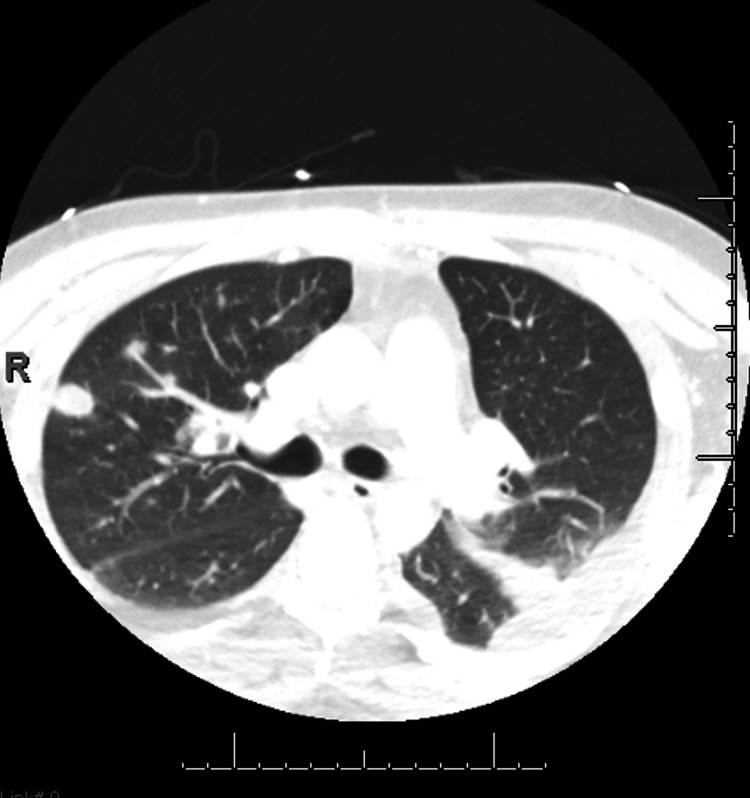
Repeat axial CT imaging showing extensive septic emboli
